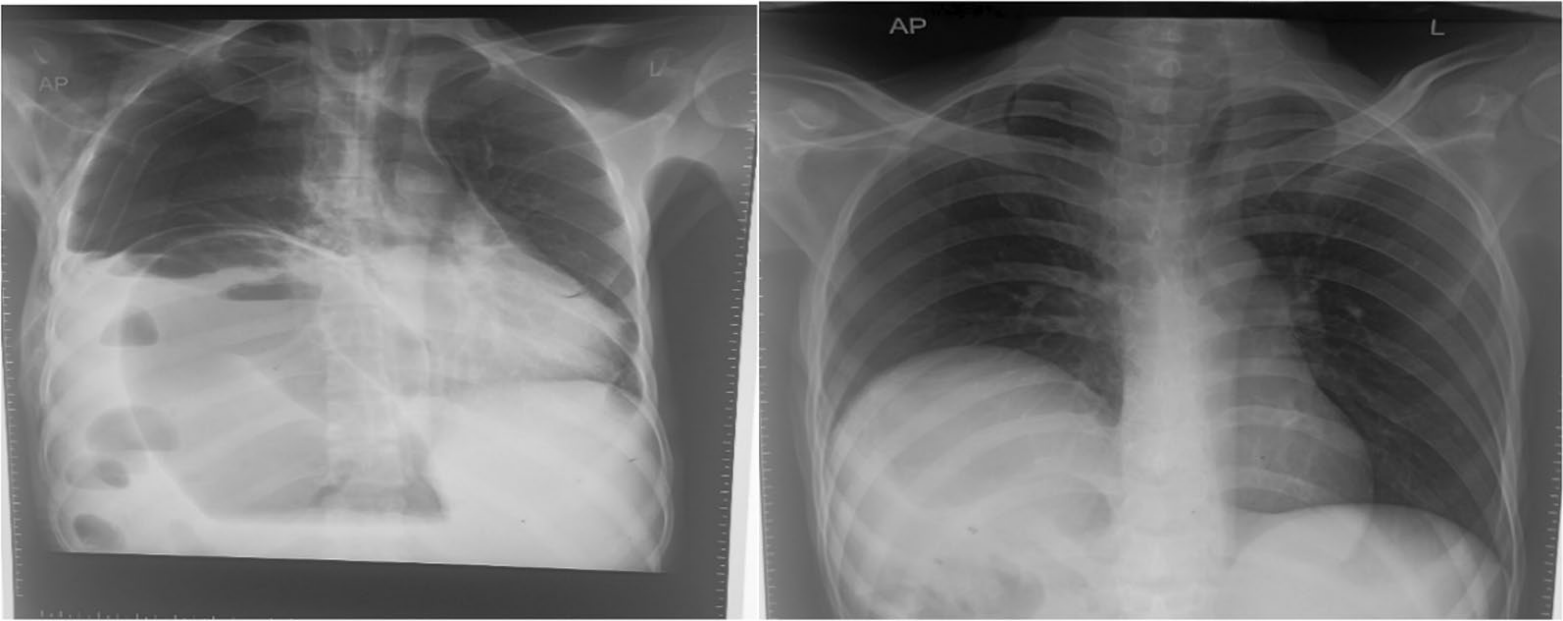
Follow-up X-rays. The image on the left shows an X-ray taken on the third postoperative day after the transverse colon was derotated and an ileostomy was placed (first operation). The image on the right shows an X-ray taken on 11 May 2022, 1 week following the transverse colon colectomy and anastomosis (second operation)
